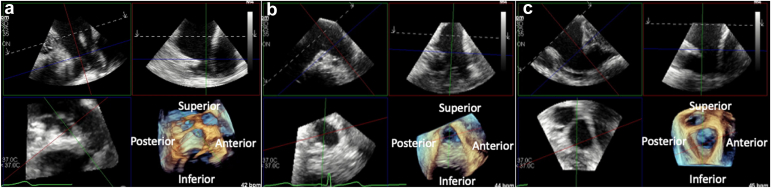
ICE 3D multiplanar reconstruction (MPR) of the LAA. Each panel corresponds to the similar panel from Figure 10. Labels are provided for spatial orientation. Panel A: mid-left atrial (LA) view. Panel B: left upper pulmonary vein (LUPV) view. Panel C: mitral valve (MV) or transmitral view Abbreviations: 3D, three-dimensional; ICE, intracardiac echocardiography; LAA, left atrial appendage.
